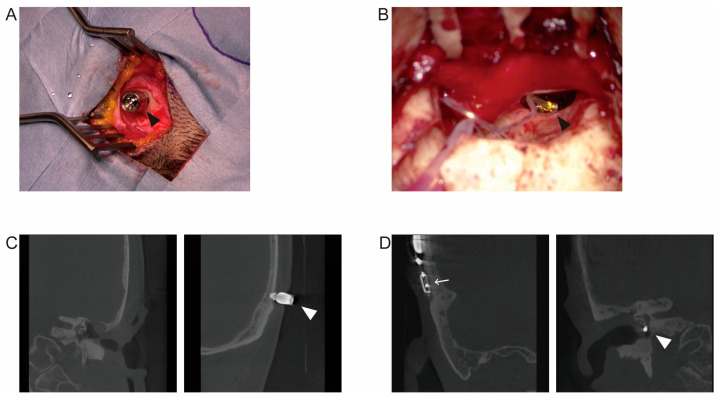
Intraoperative findings and postoperative CT images of implant insertion surgeries. (A) Observation during the Baha implant surgery. The arrowhead indicated the implant embedded in the temporal bone and the connected abutment. (B) Observation during the Vibrant Soundbridge (VSB) implant surgery. The coil was left in the cavity after a mastoidectomy and the floating mass transducer was installed in the round window niche (indicated by the arrowhead). (C) Coronal sections of a CT image following Baha implant surgery on left side. The arrowhead indicates the implant embedded in the temporal bone and the abutment. The abutment was exposed on the skin surface. (D) Coronal sections of a CT image following VSB implant surgery on right side. The implant (indicated by the arrow) was fixed to the temporal bone with screws and was buried subcuta-neously. The arrowhead indicates the floating mass transducer left in the round window niche.
